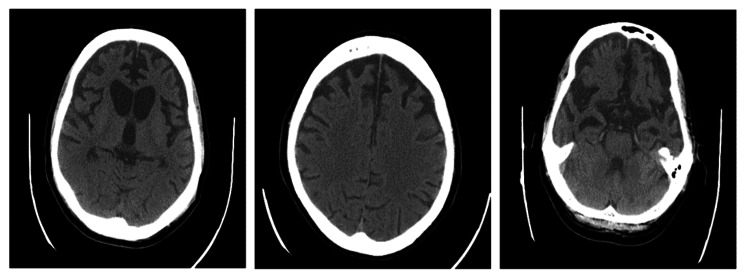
computed tomography (CT) scan: Cortical brain atrophy is more significant in the frontal, parietal, and temporal lobes. Dilatation of both lateral ventricles, especially in the frontal horn and third and fourth ventricles, suggests focal atrophy
